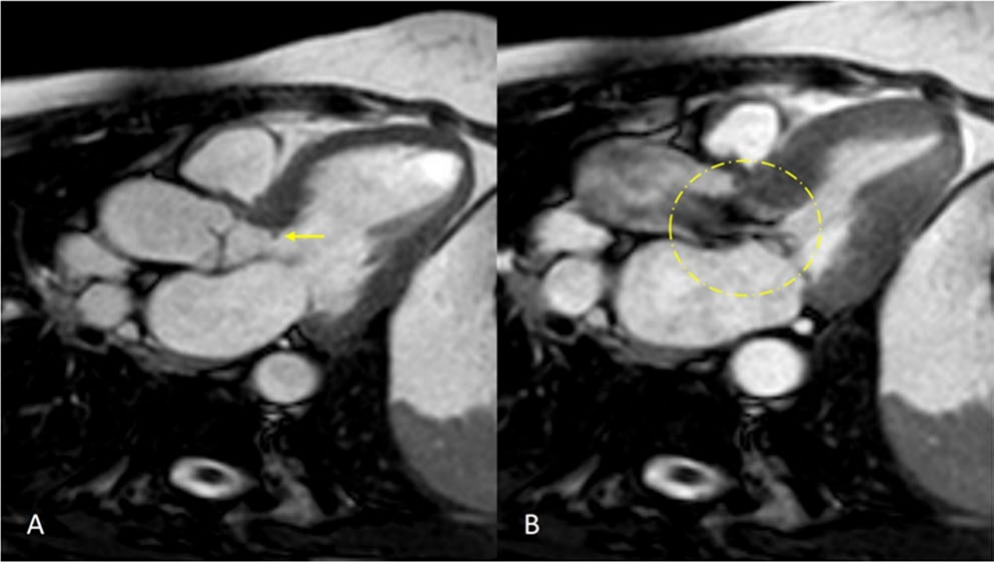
Cardiac Magnetic Resonance Imaging, three chamber view showing subaortic membrane (A, yellow arrow) with dephasing jet across left ventricular outflow during left ventricular systole (B)
